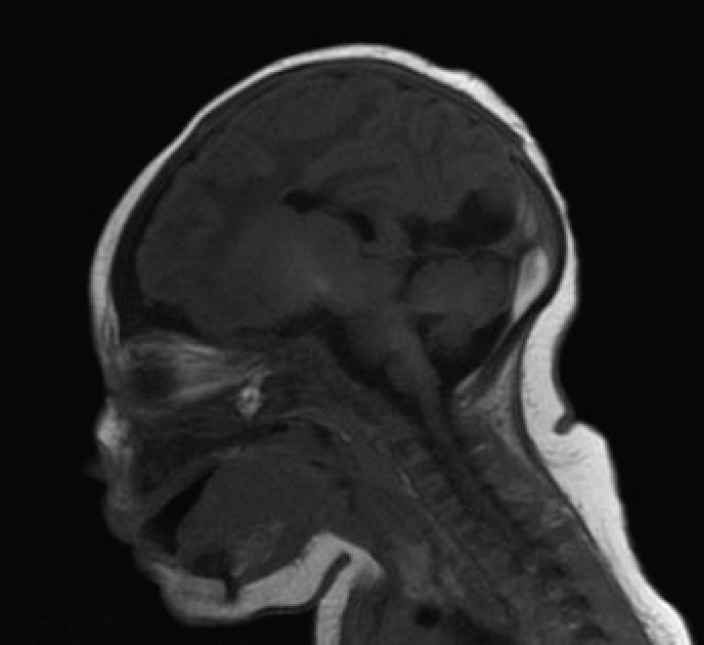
Sagittal T1 flair MRI image showing missing anterior part of the corpus callosum
